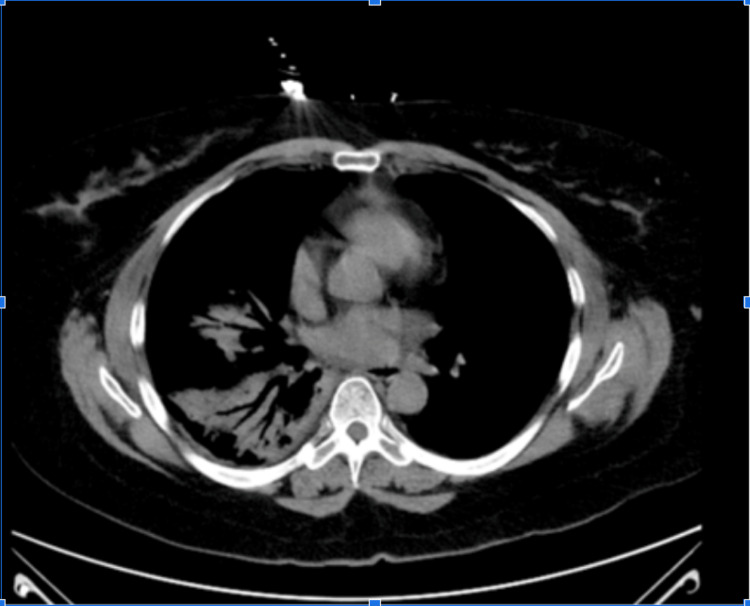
CT angiography performed on on Day 5
